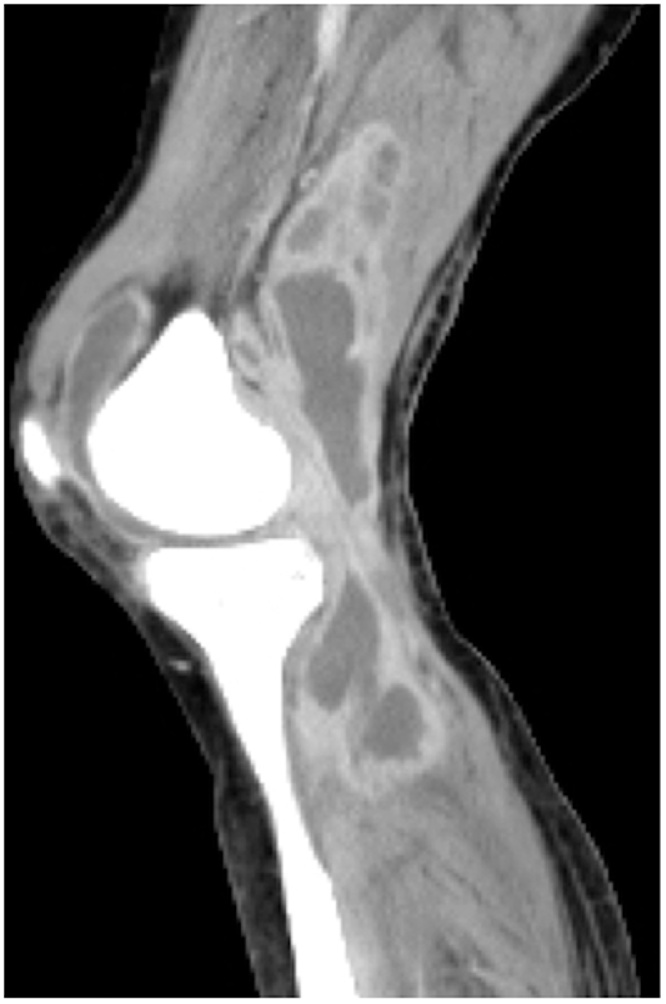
Contrast-enhanced CT of the patient’s right knee on day 22.
